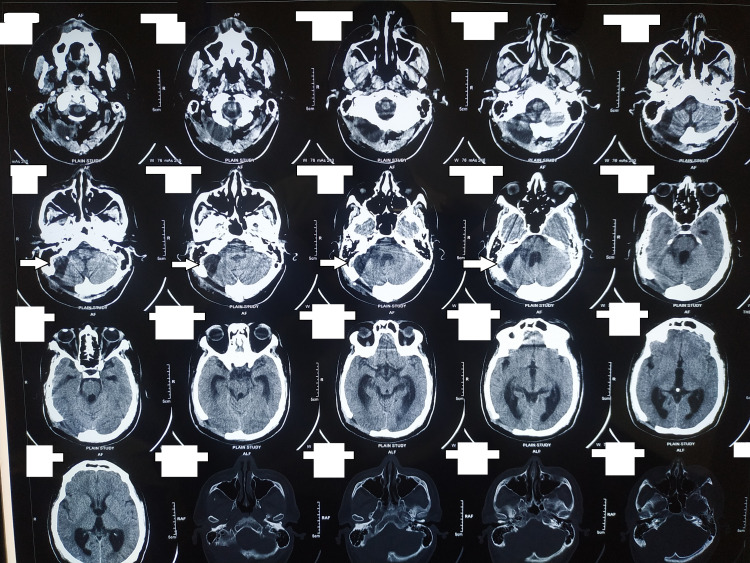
Postoperative CT scan of the brain CT: computed tomography
